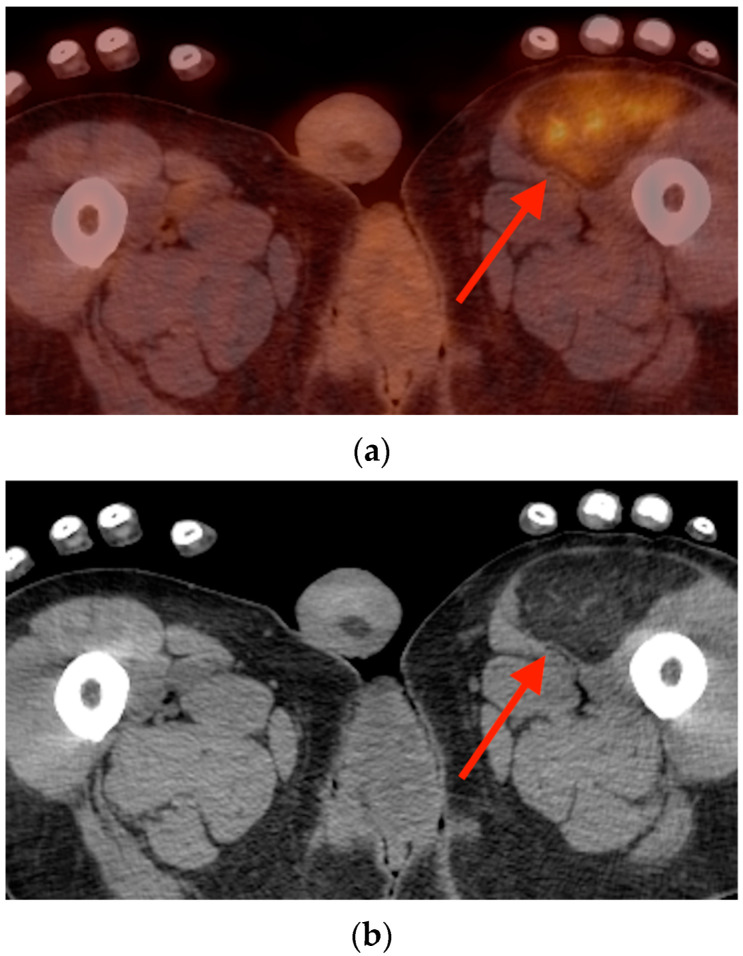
Axial fused FDG PET/CT (a) and plain CT (b) images obtained in a 54-year-old man with bladder cancer show an area of FDG uptake in the anterior left thigh, SUVmax 5.9, which corresponds with a well-circumscribed fat density lesion (red arrows). This was later biopsied, with pathology revealing a hibernoma. CT = computed tomography. FDG = fluorine-18 fluorodeoxyglucose. PET/CT = positron emission tomography/computed tomography.
